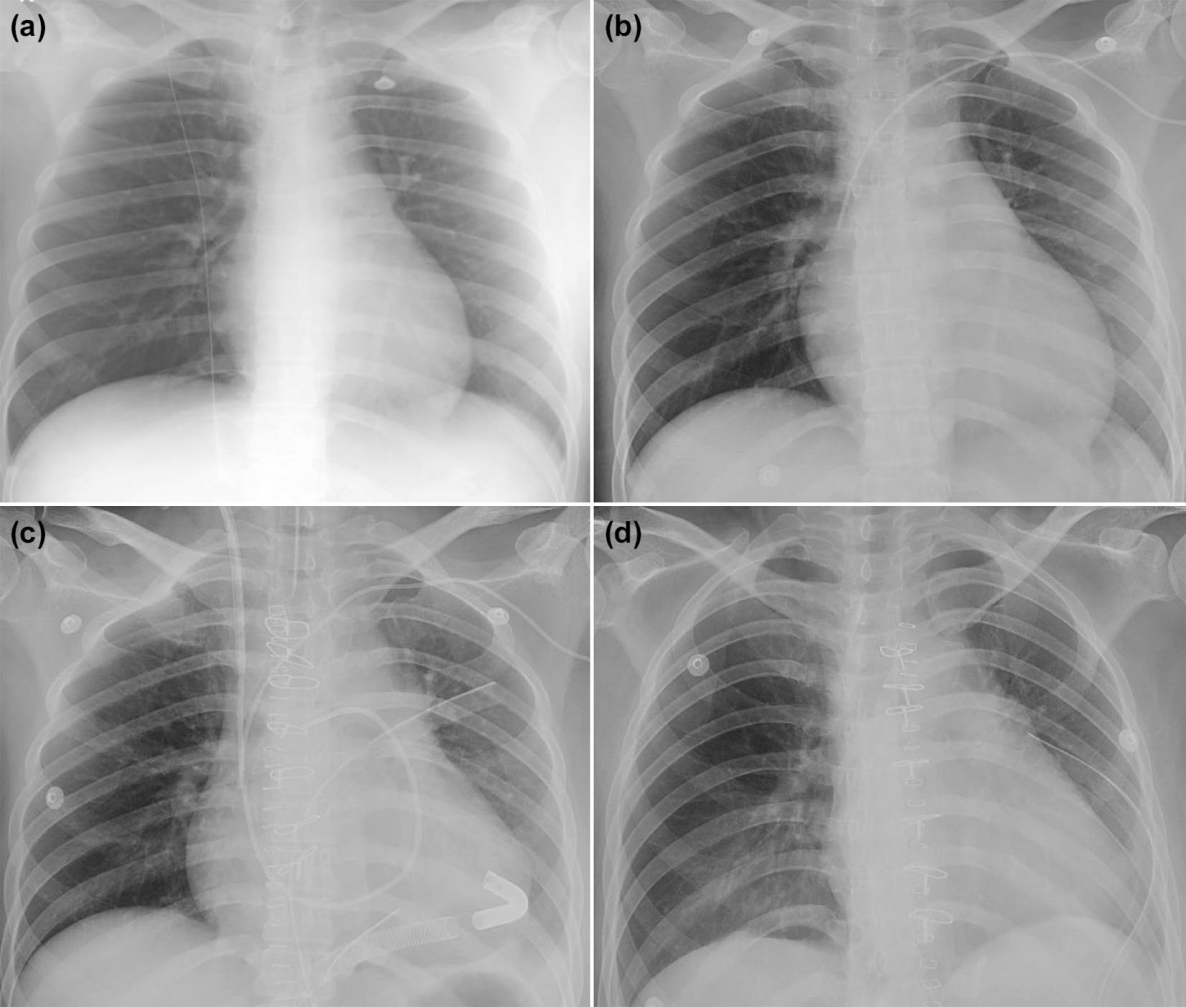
Representative postpartum patient who received ECMO. A 34-year-old pregnant woman with a fetus with a gestational age of 16 weeks was diagnosed with breast cancer stage IA, underwent right total mastectomy, and received adjuvant doxorubicin and cyclophosphamide chemotherapy. She delivered at a gestational age of 36 weeks and was discharged without significant symptoms (a). After 3 weeks, she presented to the emergency room with dyspnea and palpitations (b). An echocardiogram revealed decreased biventricular systolic function with an LVEF of approximately 25%. After 2 weeks of intensive care unit management with dobutamine and volume control, her symptoms did not improve. Emergency extracorporeal LVAD insertion was performed, and 8 days after extracorporeal LVAD insertion (c), she underwent heart transplantation (d). *ECMO* extracorporeal membrane oxygenation, *LVEF* left ventricle ejection fraction, *LVAD* left ventricular assist device.
